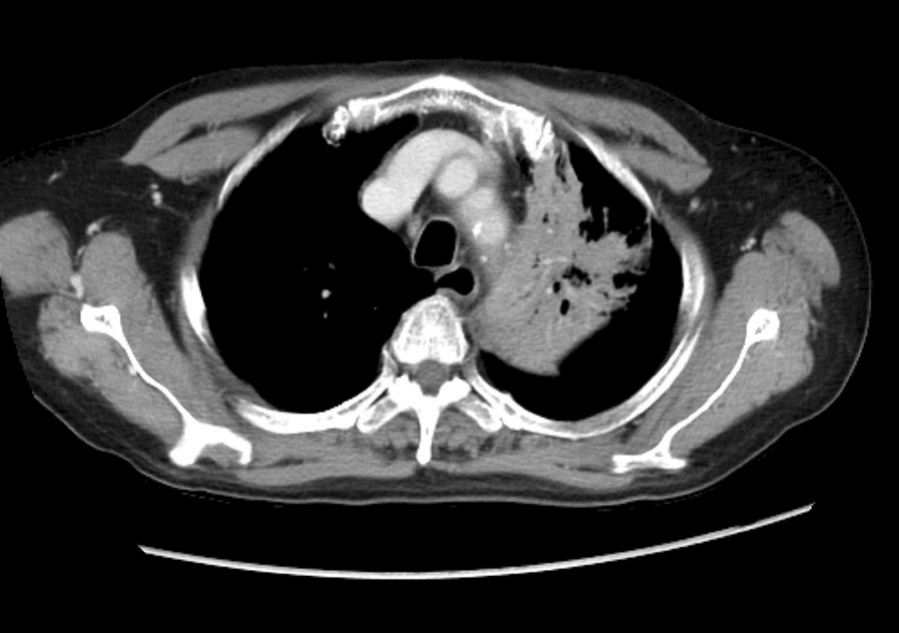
The chest CT shows left upper lung consolidation
